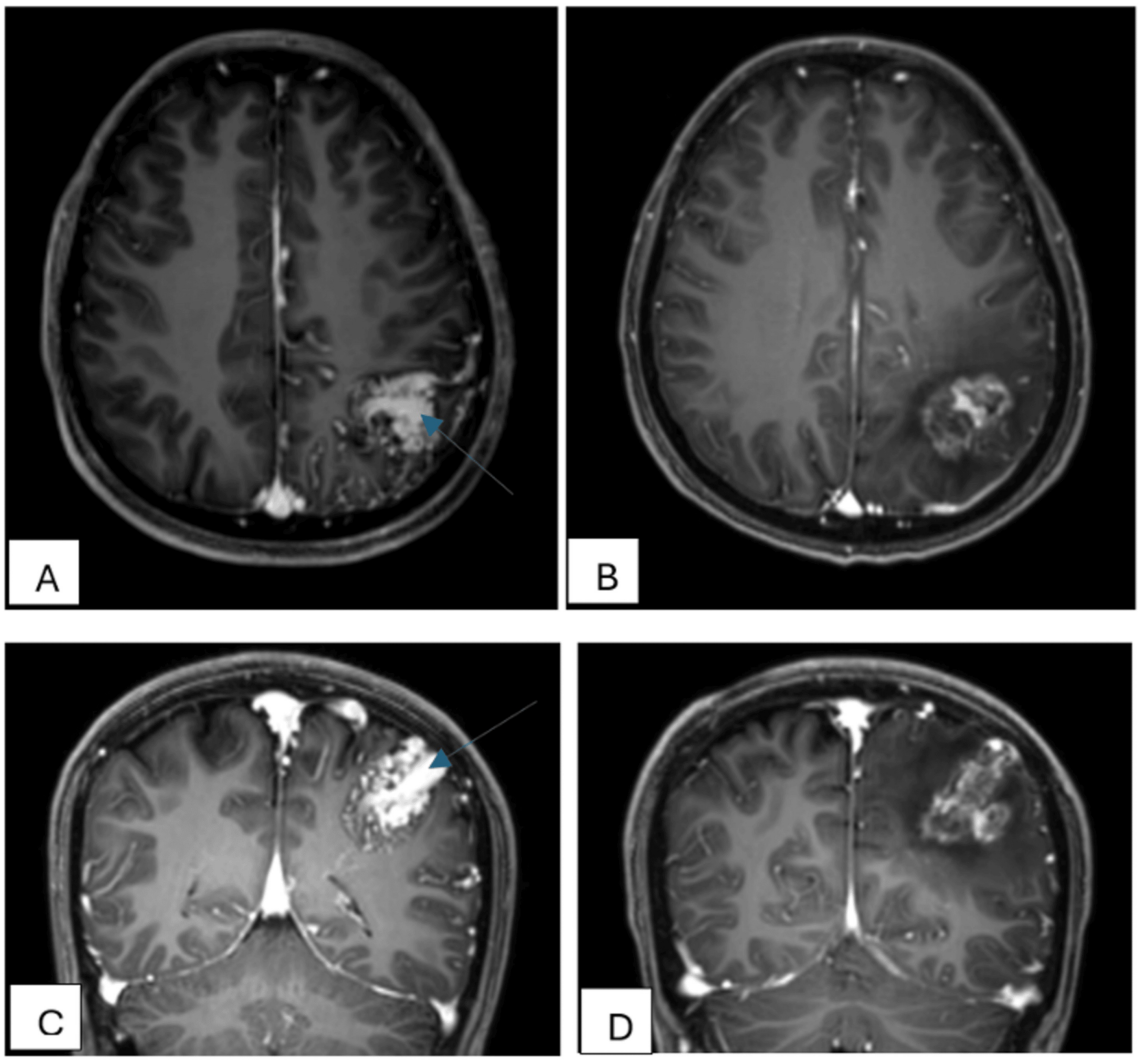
MRI brain image pre-SRS in (A) axial and (C) coronal showing nidus with large feeding arteries (blue arrow) as comparison with images of MRI brain two years after intervention (SRS) on (B & D) showing obliteration of the feeding arteries (not seen). MRI images post-intervention also show the necrosis changes of the nidus from the radiation given with regions of perilesional oedema. SRS: Stereotactic radiosurgery
